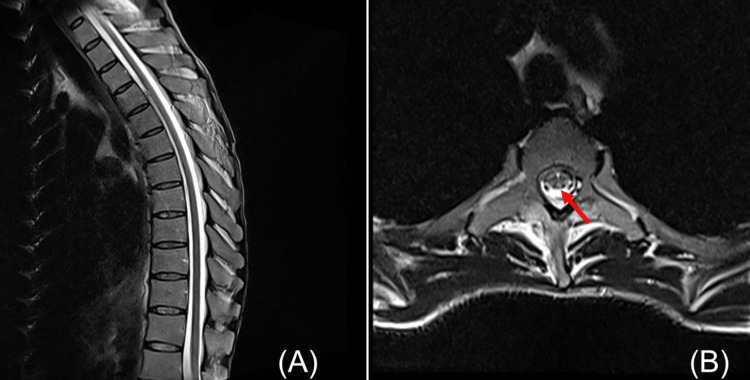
Spinal cord MRI (T2-weighted images). (A) Sagittal T2-weighted image of the thoracic spine showing no apparent abnormal signal intensity. (B) Axial T2-weighted image at the mid-to-lower thoracic level (approximately T8–T9) demonstrating bilateral symmetric high-signal intensity areas along the posterior columns of the spinal cord. Images were obtained using a 1.5-T scanner with standard spinal imaging protocols.
